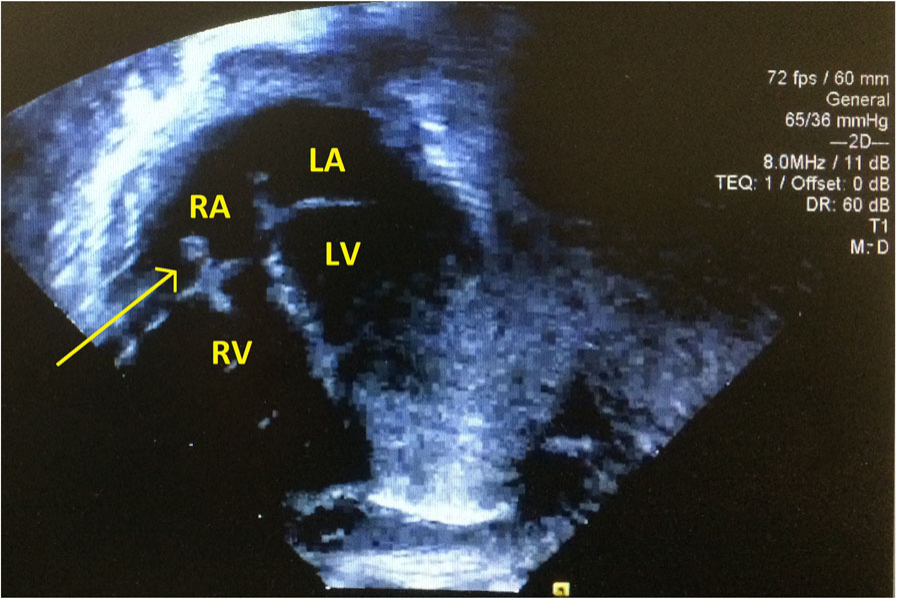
Apical 4-chamber view on echocardiograph showing a 0.40-cm vegetative lesion on the tricuspid valve (indicated by arrow). RA = right atrium, RV = right ventricle, LA = left atrium, LV = left ventricle
